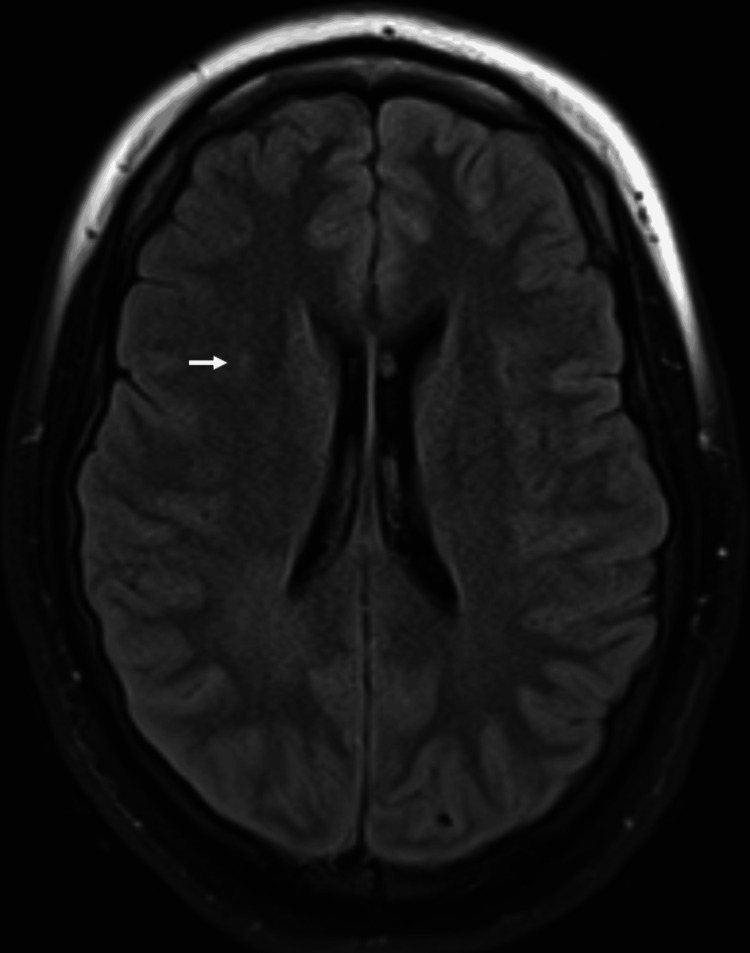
Brain MRI A brain MRI reveals a subtle increased FLAIR signal in the right periventricular white matter, as indicated by the white arrow. FLAIR: fluid-attenuated inversion recovery
